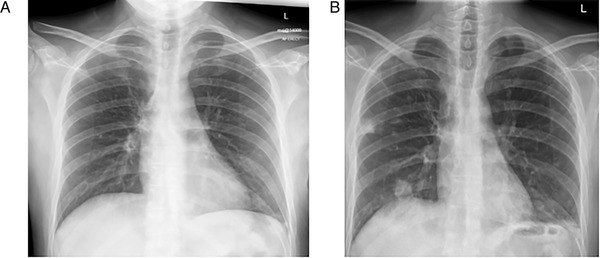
The progression of chest X‐ray changes. (A) Chest radiograph on admission and (B) with increasing oxygen requirement
